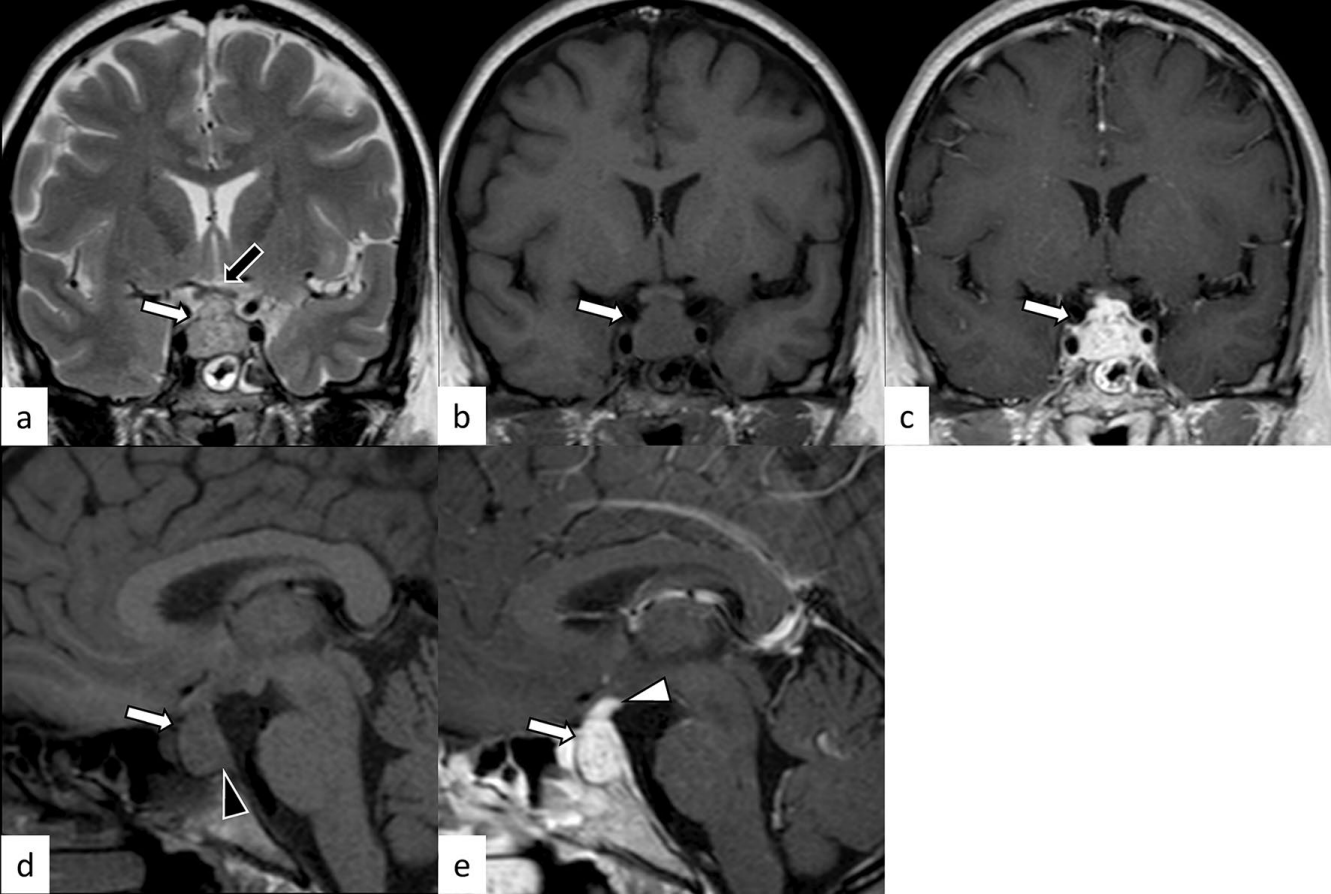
A 45-year-old woman with secondary granulomatous hypophysitis associated with Crohn’s disease. Both pituitary gland and stalk are enlarged (a–e, white arrows; e, white arrowhead), abutting the optic chiasm superiorly (a, black arrow). The pituitary gland shows heterogeneous hyperintensity on T2-weighted coronal image (a), hypointensity on T1-weighted coronal image (b), and heterogeneous vivid enhancement (c, e). The posterior pituitary T1-weighted bright spot is absent (d, black arrowhead)
